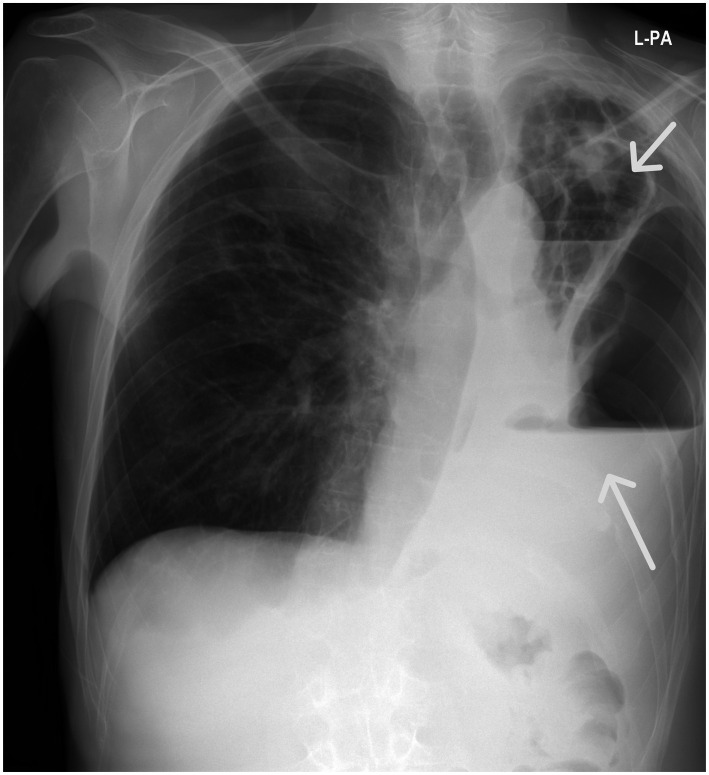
Chest X-ray (posterior–anterior position): Bilateral excavated lung lesions with left-sided hydropneumothorax.
